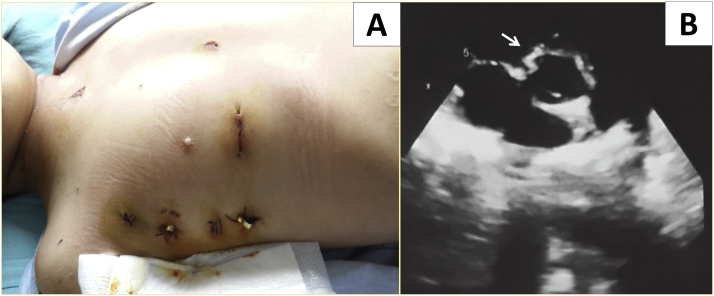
(A): Surgical scars prior to discharge; (B): Artificial patch (white arrow) in parasternal short axis view.
